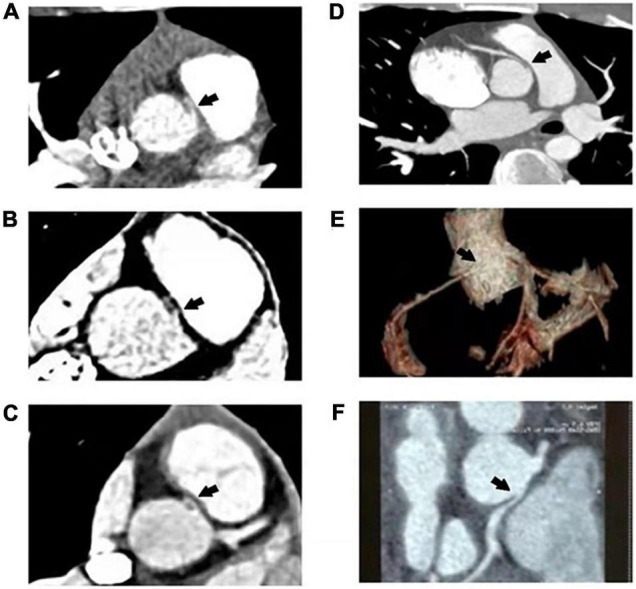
Computed tomography angiography (CTA) of six patients. Black arrows indicate the interarterial course between the aorta and pulmonary artery. (A) Interarterial R-ACAOS with no obvious stenosis (Patient 1). (B) Interarterial R-ACAOS with proximal RCA stenosis (Patient 2). (C) Interarterial R-ACAOS with no obvious stenosis (Patient 4). (D) Interarterial R-ACAOS with proximal RCA stenosis (Patient 6). (E) Interarterial R-ACAOS with proximal RCA stenosis (Patient 7). (F) Interarterial L-ACAOS with proximal LCA stenosis (Patient 8).
